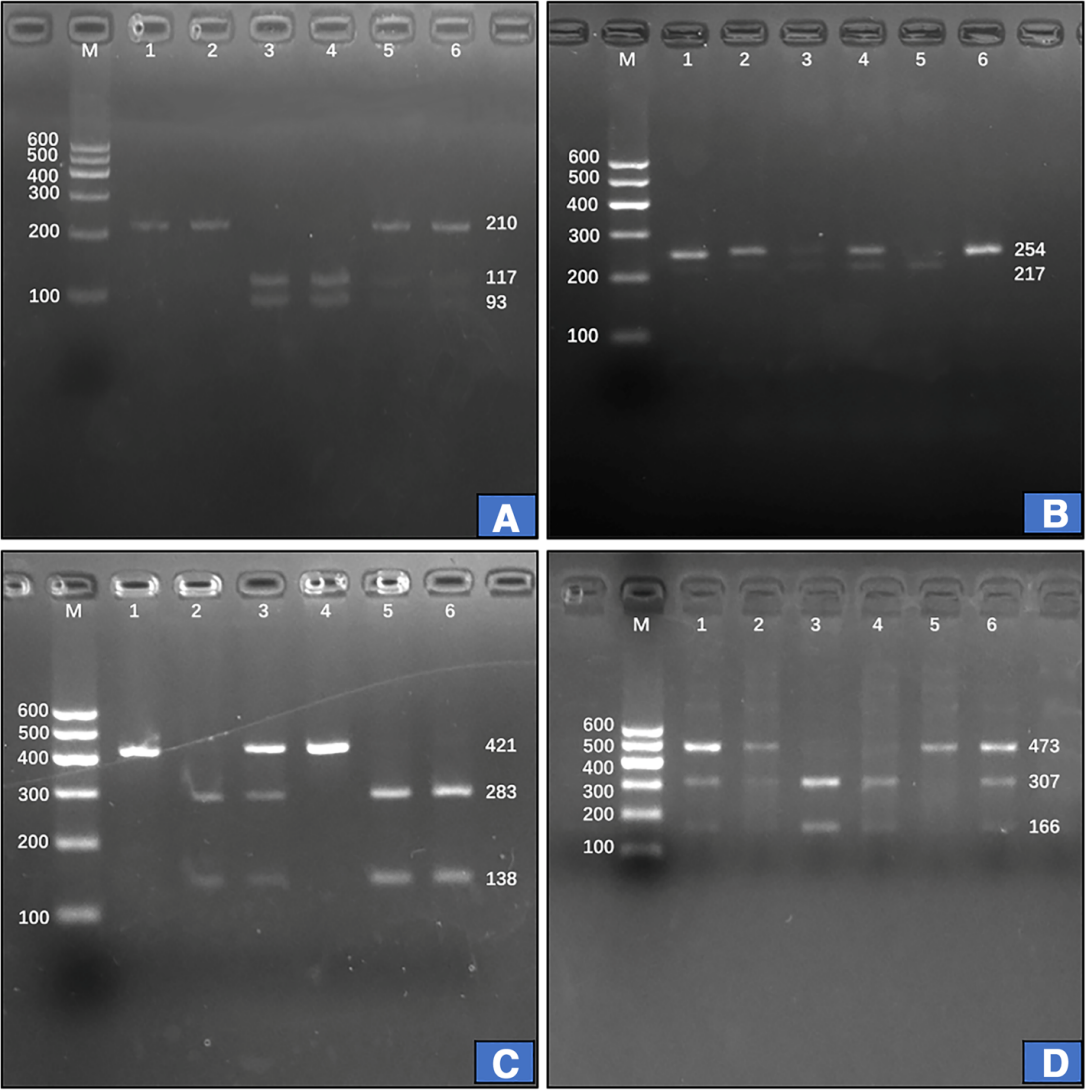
Genotyping of the four *LIPG* SNPs. Lane M is the 100 bp marker ladder; lanes 1–6 are samples. a (rs3813082): lanes 1 and 2, GG genotype (210 bp); lanes 3 and 4, TT genotype (117- and 93-bp); and lanes 5 and 6, GT genotype (210-, 117- and 93-bp); b (rs2000813): lanes 2 and 6, CC genotype (254-bp); lanes 3 and 4, CT genotype (254-, 217- and 37-bp); and lanes 1 and 5, TT genotype (254- and 217-bp); c (rs2097055): lanes 1 and 4, CC genotype (421-bp); lanes 2, 5 and 6, TT genotype (283- and 138-bp); and lane 3, CT genotype (421-, 283- and 138-bp); d (rs3819166): lanes 2 and 5, GG genotype (473-bp); lanes 3 and 4, AA genotype (307- and 166-bp); and lanes 1 and 6, AG genotype (473-, 307- and 166-bp). The bands less than 90-bp fragments were not visible in the gel owing to their fast migration speed
